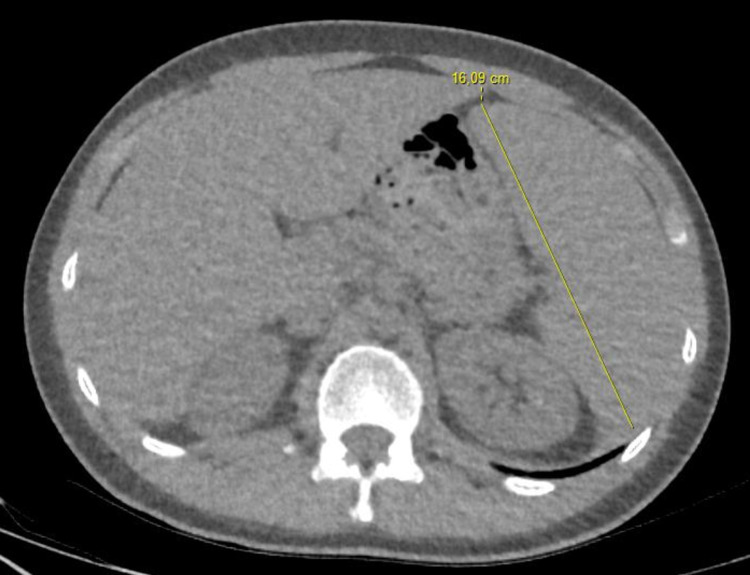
CT image of patient with hepatosplenomegaly.
